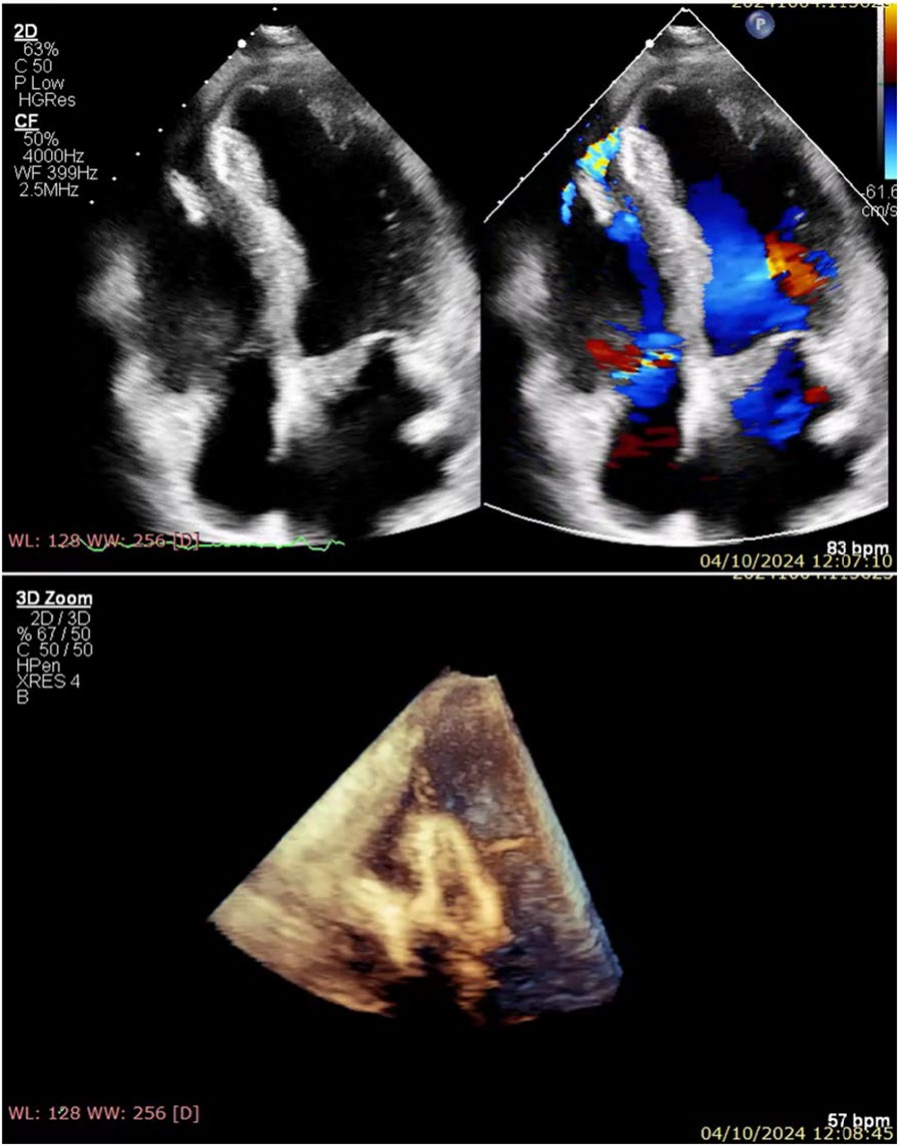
One-month follow-up echocardiographic evaluations confirmed that the occluder was properly positioned, with minimal residual flow
